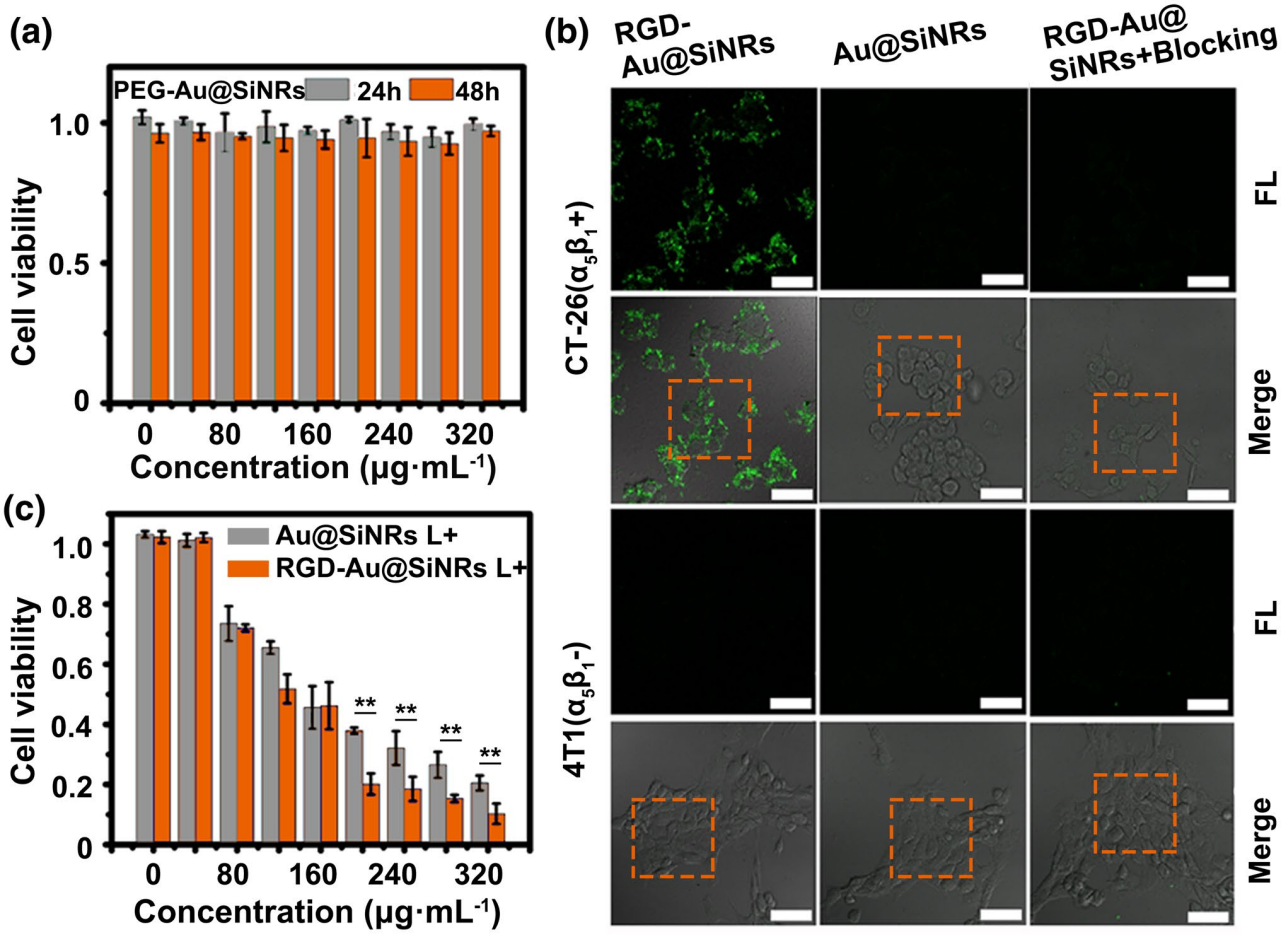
Assessment of the biocompatibility, targeted imaging, and photothermal effect in vitro. a Cytotoxicity of PEG-Au@SiNRs. b LSCM images of CT-26 and 4T1 cells after incubation with RGD-Au@SiNRs (blocking with free peptides or not) or Au@SiNRs for 2 h at 37 °C. Scale bars, 25 μm. c Cell viability of CT-26 cells, which were first incubated with RGD-Au@SiNRs or Au@SiNRs for 4 h and then irradiated by an 808-nm laser (0.8 W cm^−2^) for 5 min as mean ± SD (*n* = 3). Asterisk () indicates *p *< 0.01
